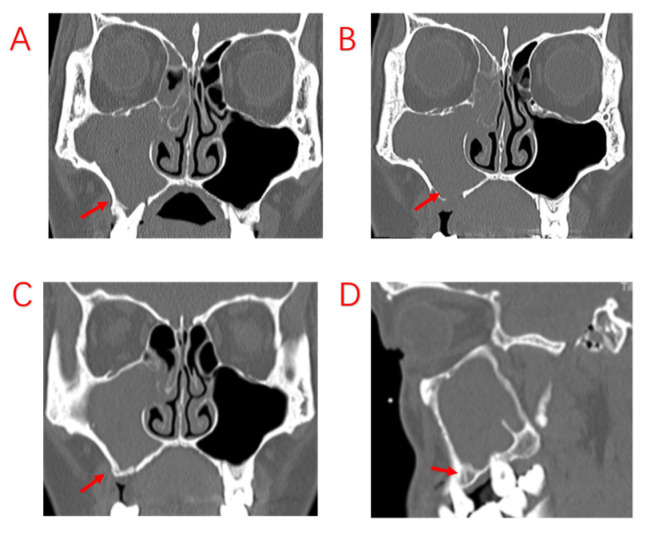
Patient undergoes tooth extraction but no sinus ESS. (A): The patient was found to have sinusitis of the right maxillary sinus at the time of tooth extraction, and the affected tooth was extracted without an oral maxillary sinus fistula. (B): The sinus CT was repeated 1 month after tooth extraction, which revealed ongoing sinus inflammation. (C,D): One year after tooth extraction, there was already new bone formation on the floor of the maxillary sinus; however, the sinus symptoms were still not relieved as the maxillary sinus was not opened. Red arrows indicate bony defects in MSF and restitution.
